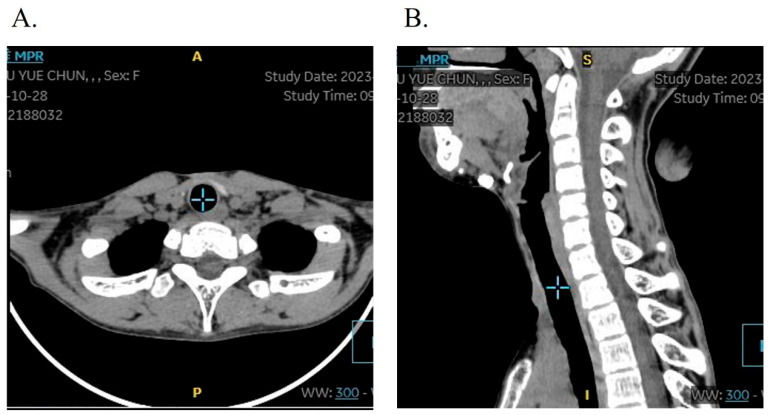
Follow-up CT of the tracheal tract in axial view (A) and sagittal view (B). There are no obvious structural changes to the trachea lumens.
